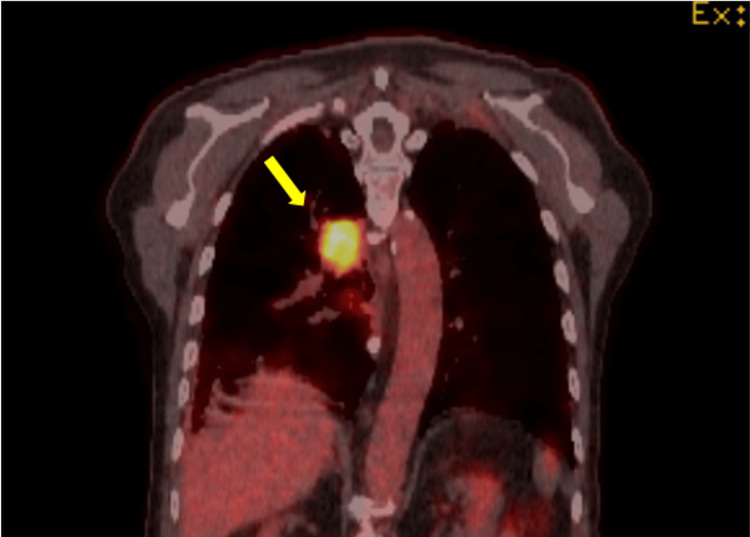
FDG PET-CT demonstrating a metabolically active lesion in the right lung (yellow arrow), consistent with a new primary pulmonary malignancy. FDG PET-CT: fluorodeoxyglucose positron emission tomography combined with computed tomography.
